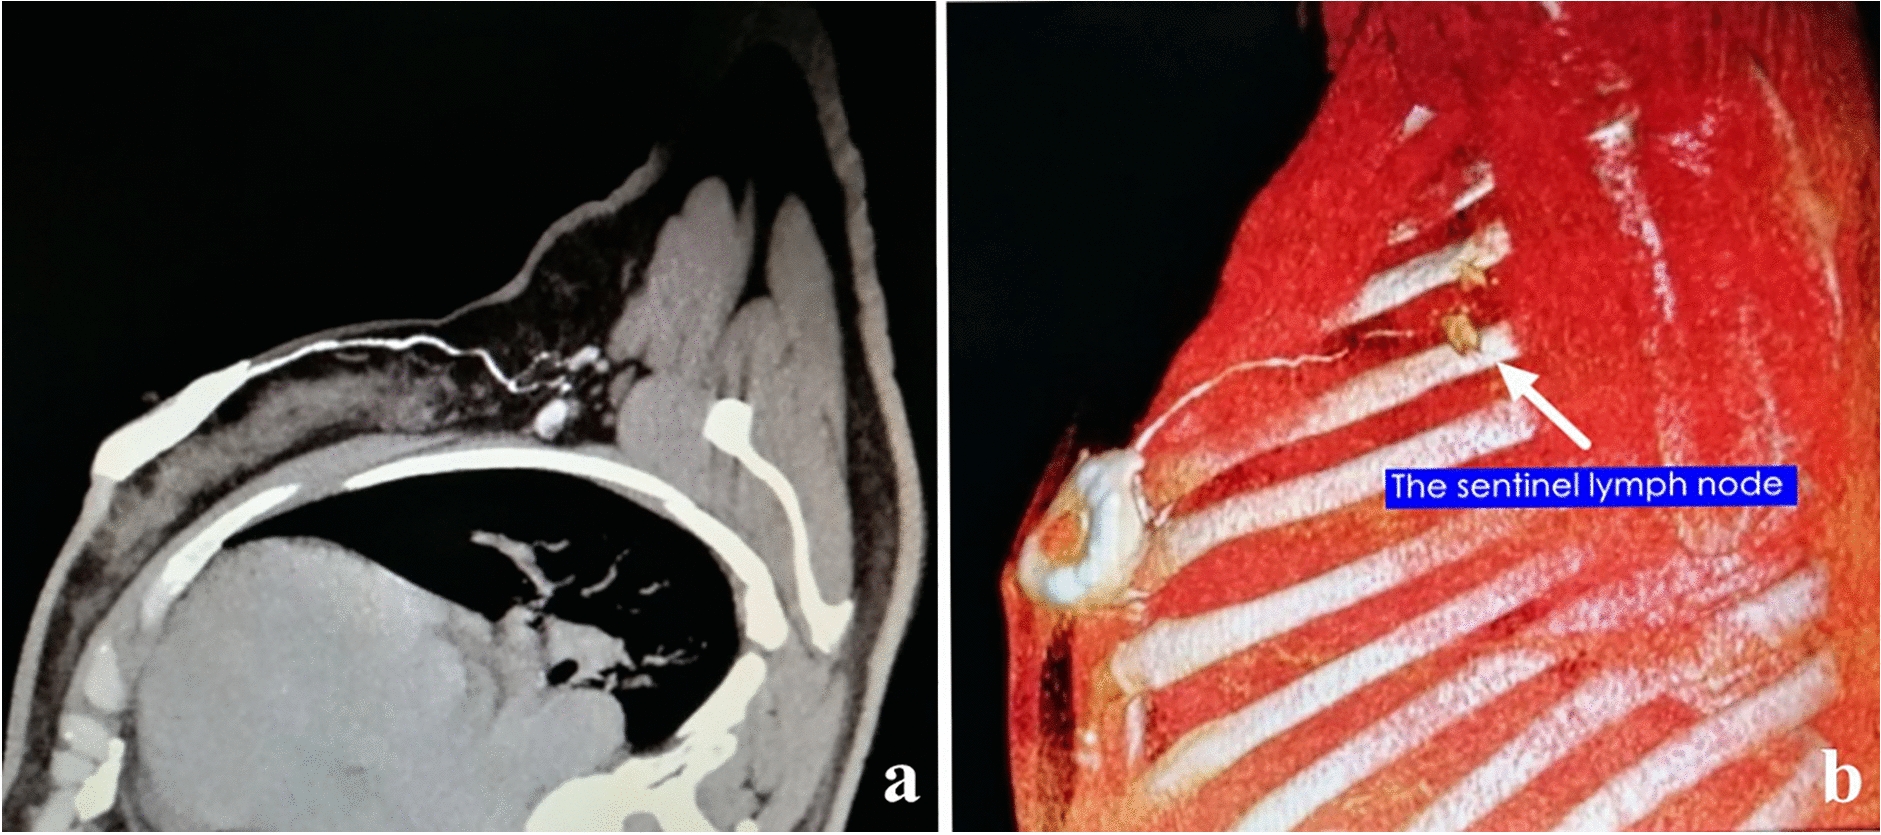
Three-dimensional CT lymphography. a CT-LG provided a clear visualization of the lymphatic vessels and sentinel lymph nodes in the axillary region. b The sentinel lymph node (white arrow) was located on the reconstructed digital 3D model
